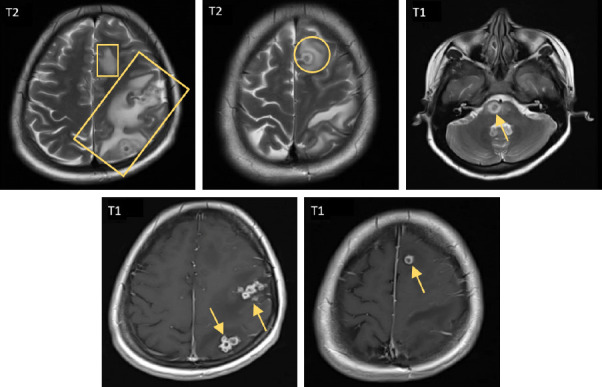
MRI head with contrast: numerous clusters of ring-enhancing lesions are noted in the left frontoparietal lobes and the right side of the pons (yellow arrows) on T1-weighted postcontrast images. The largest lesion, measuring 8 mm in diameter, demonstrates target sign with central low signal intensity and surrounding vasogenic edema (yellow circle) on T2-weighted image. Area of vasogenic edema is also seen in the left parietal region related to other similar lesions (yellow rectangles) on T2-weighted image. No definite abnormal meningeal enhancement is seen.
